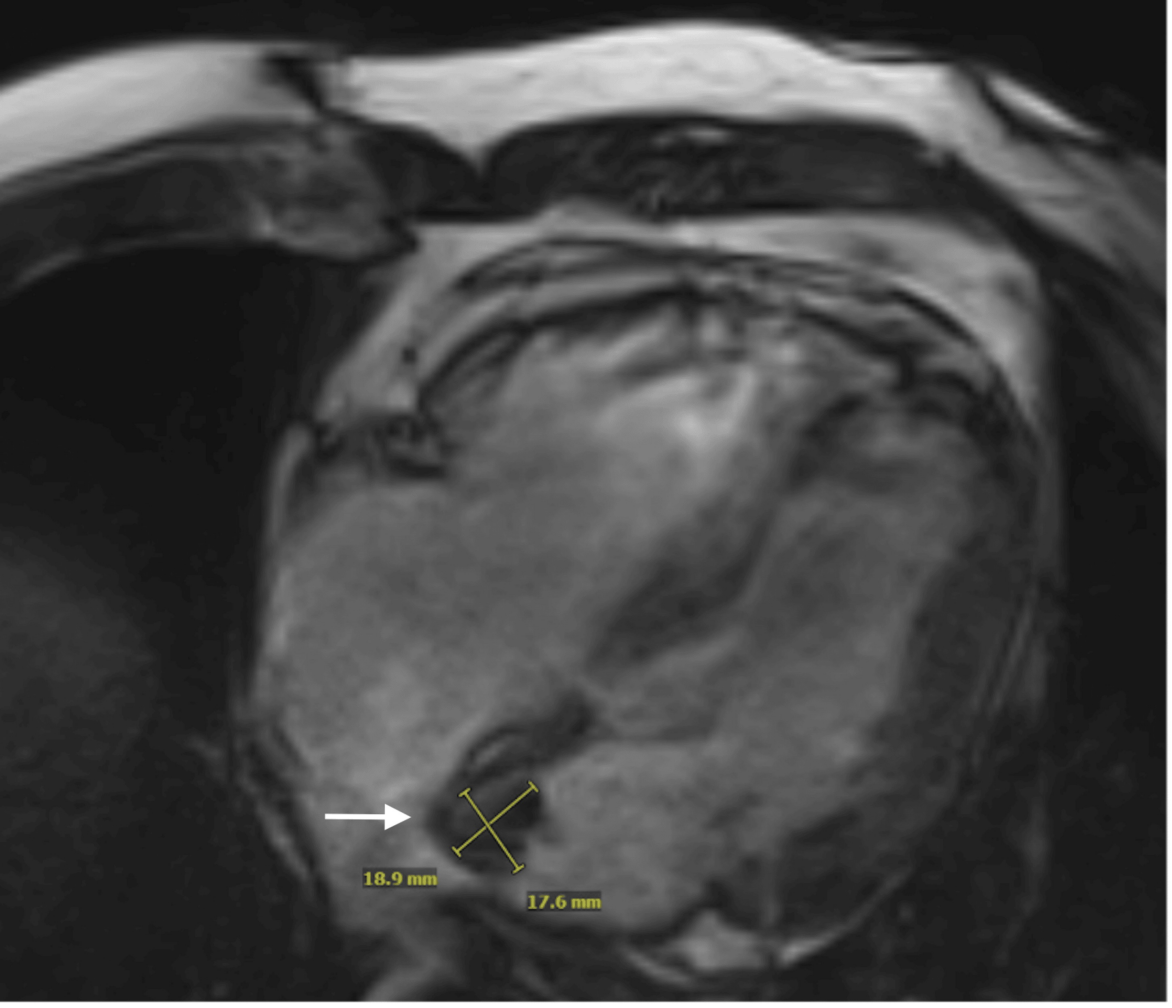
Cardiac MRI An 18.9 × 17.6 mm mass (arrow) in the left atrium abutting the interatrial septum was observed, with no definite enhancement, and demonstrated low T1 and intermediate-to-high T2 signals.
